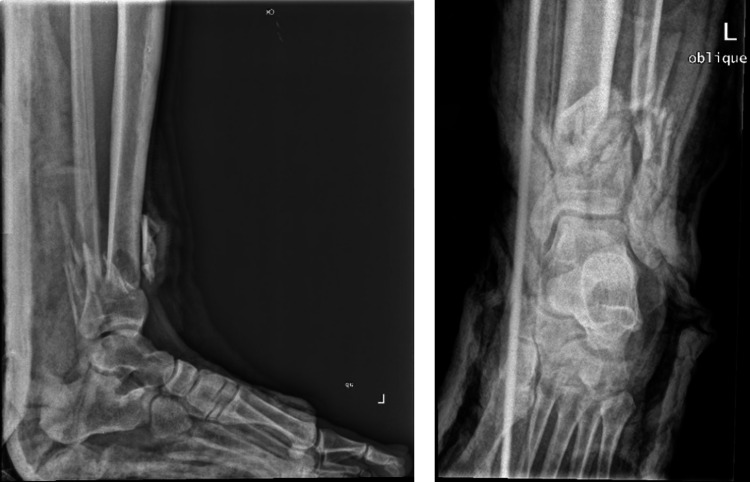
Initial anteroposterior and lateral x-rays showing excessive comminution at the fracture site.
